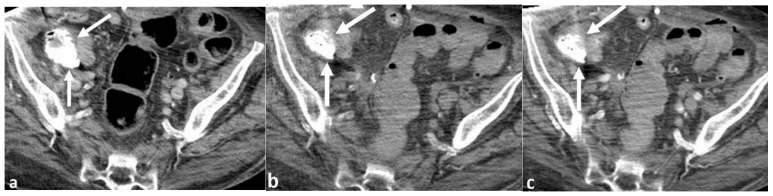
A 72-year-old woman with rectal bleeding. Axial intravenous contrast-enhanced image from single phase CT (a) shows hyperattenuating contents in the cecum (arrows), which was interpreted as active bleeding. Non-IV contrast (b) and arterial phase (c) images from the CT angiogram performed one day later again shows hyperattenuating contents (arrows), similar in volume and distribution to the previous CT scan and without change between the two series, proving that the hyperattenuating material was ingested material rather than intravenous contrast extravasation.
